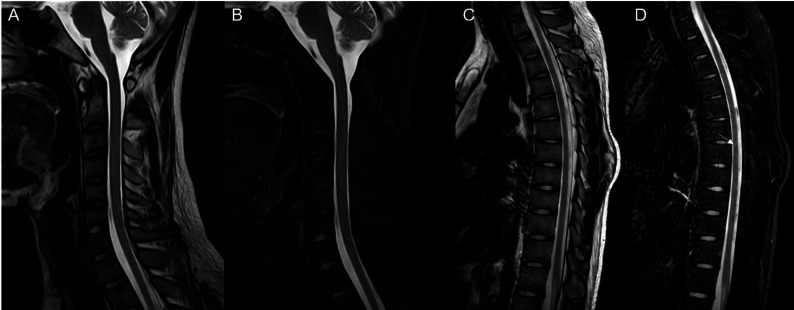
Cervical and thoracic spine MRI after treatment: Fat-suppressed images (D) demonstrate that the lesion at the T6–7 level has markedly decreased in extent, with no definite abnormal signal now visible at any previously affected segment (A, B, C)
